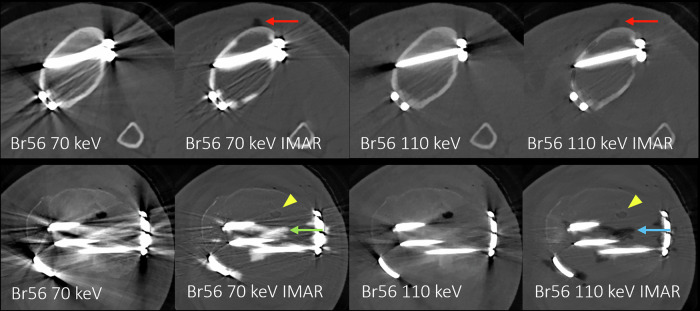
Axial CT images showing artifacts and loss of anatomical information in iMAR and high-VMI images. Images were reconstructed using a Br56 kernel with 0.4 mm slice thickness, with and without iMAR at 70 and 110 keV. A low-attenuating artifact adjacent to the tibia appears (red arrow), and a gas bubble within the tibia is almost eliminated (yellow arrowhead) in iMAR images. Hyperattenuating artifacts are enhanced at 70 keV with iMAR (green arrow) and are converted into low-attenuating artifacts at 110 keV with iMAR (blue arrow)
